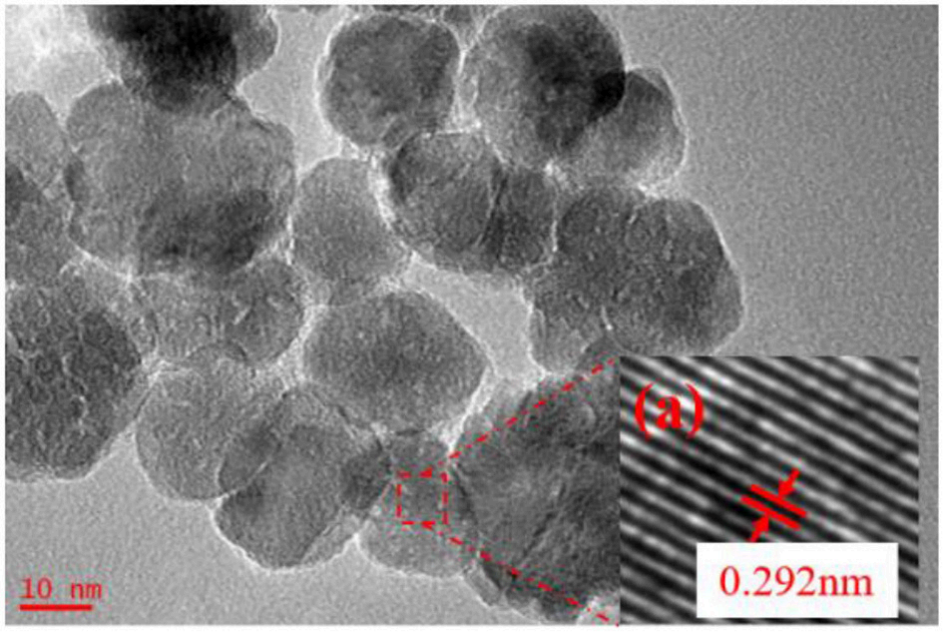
HRTEM images of ZrO_2_ materials synthesized with 5 min radiation time at ultrasonic power of 90% and when the molar ratio of precursor to precipitant is 1:1.
